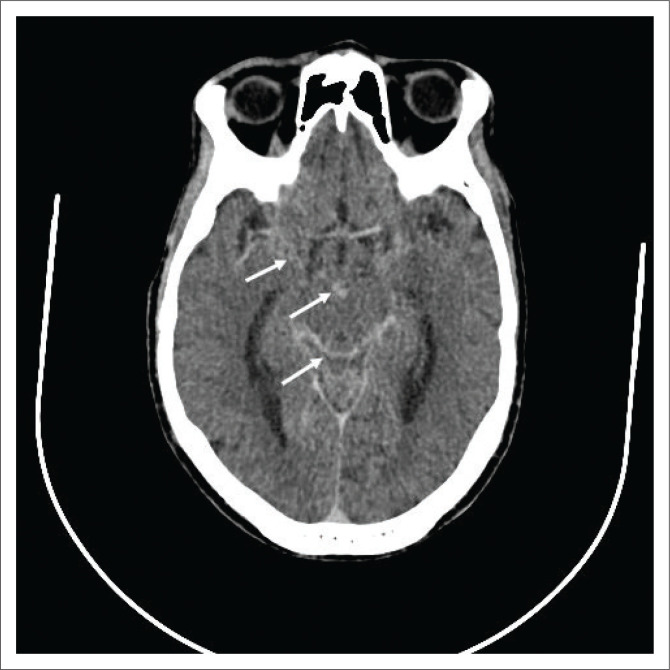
A 50-year-old patient with human immunodeficiency virus presented with new onset seizures and a reduced Glasgow coma scale (11/15). Post-contrasted computed tomography of the brain revealed diffuse leptomeningeal enhancement (white arrows) consistent with tuberculosis meningitis, which was confirmed based on cerebrospinal fluid analysis.
